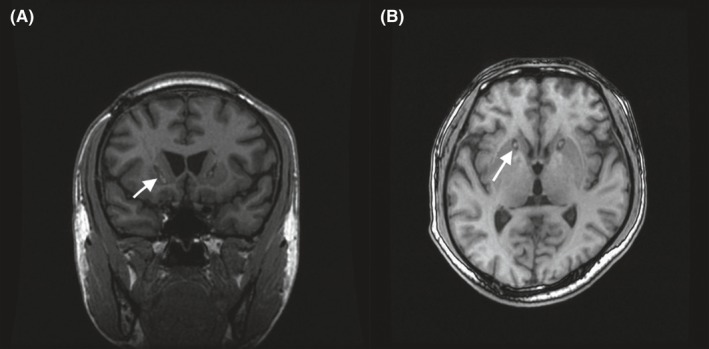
6‐A: T1‐weighted coronal sequence. 6‐B: T1‐weighted axial sequence. Postoperative magnetic resonance images showing the lesions located in the anterior capsule. A, T1‐weighted coronal sequence, and (B) T1‐weighted axial sequence showing the lesions (white arrows)
